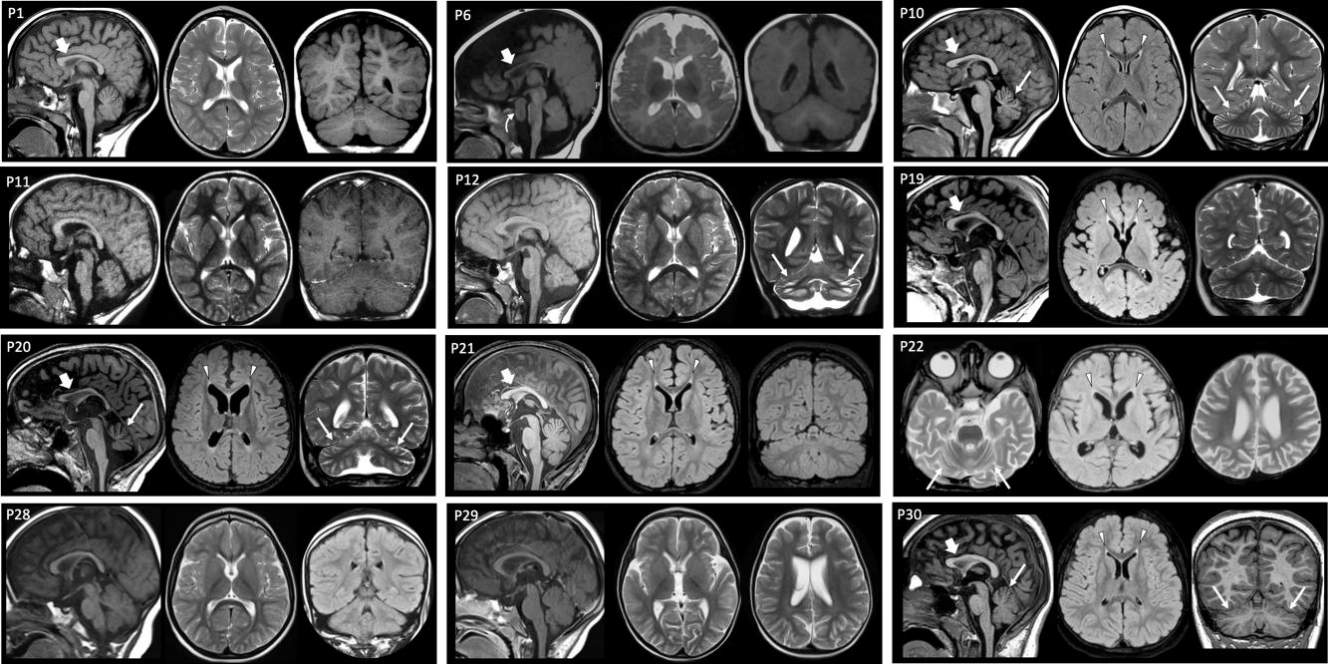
Neuroimaging features of RBL2-related disorder. Sagittal T_1_-weighted image (*left*), axial T_2_-weighted or FLAIR image (*middle*) and coronal T_1_- or T_2_-weighted or FLAIR image (*right*). Most subjects have an enlargement of the cerebral CSF spaces, with an anteroposterior gradient associated with thinning of the corpus callosum (thick arrows), particularly in the anterior portions. There is additional cerebellar atrophy in P10, P12, P20, P22 and P30 (thin arrows). Bilateral mild-to-moderate signal changes are noted at the level of the forceps minor in P10, P19, P20, P21, P22 and P30 (arrowheads). Note the large prepontine lesion in P6 (curved arrow). FLAIR = fluid-attenuated inversion recovery; P = patient.
